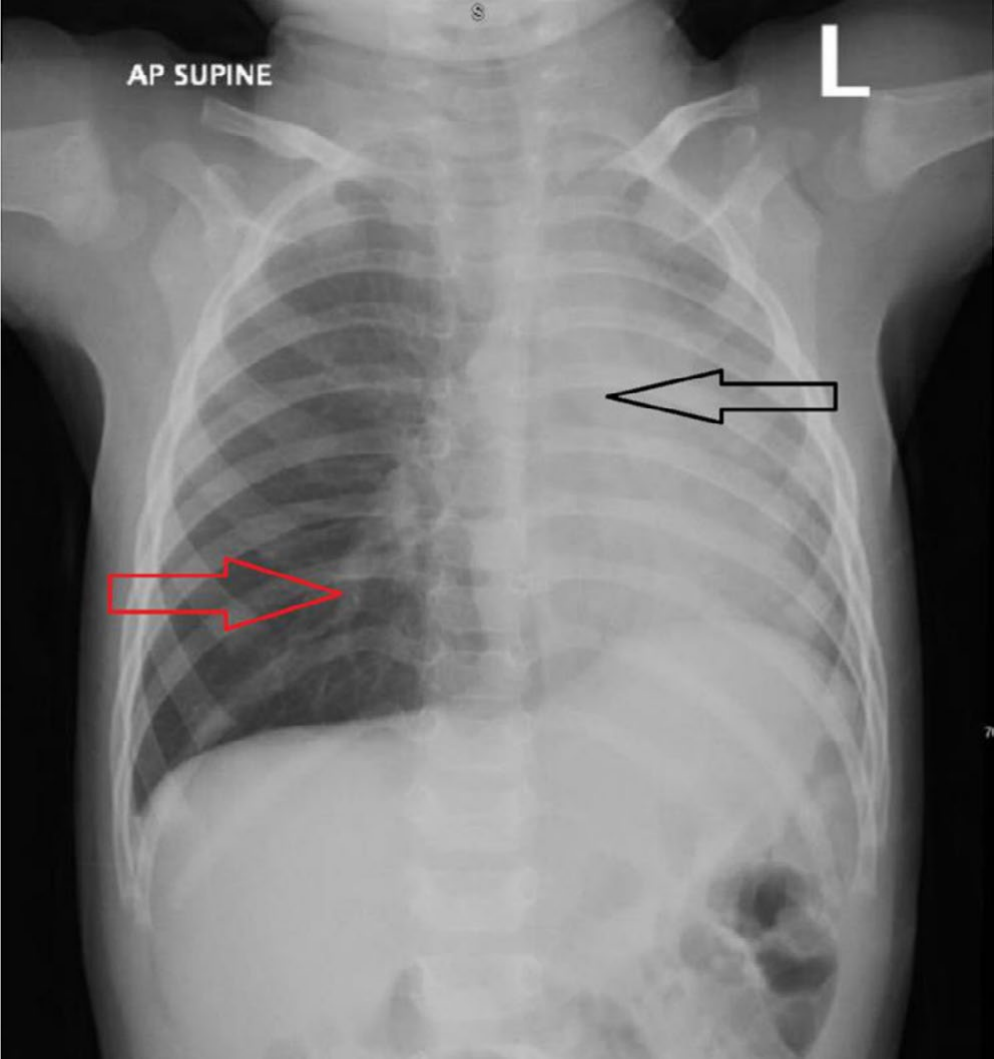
Anteroposterior (AP) supine chest radiograph of a pediatric patient on admission. The black arrow highlights hyperinflation in the right lower lobe of the lung, whereas the red arrow indicates opacity in the left lower lobe, suggestive of potential pathological changes such as pneumonia or atelectasis.
